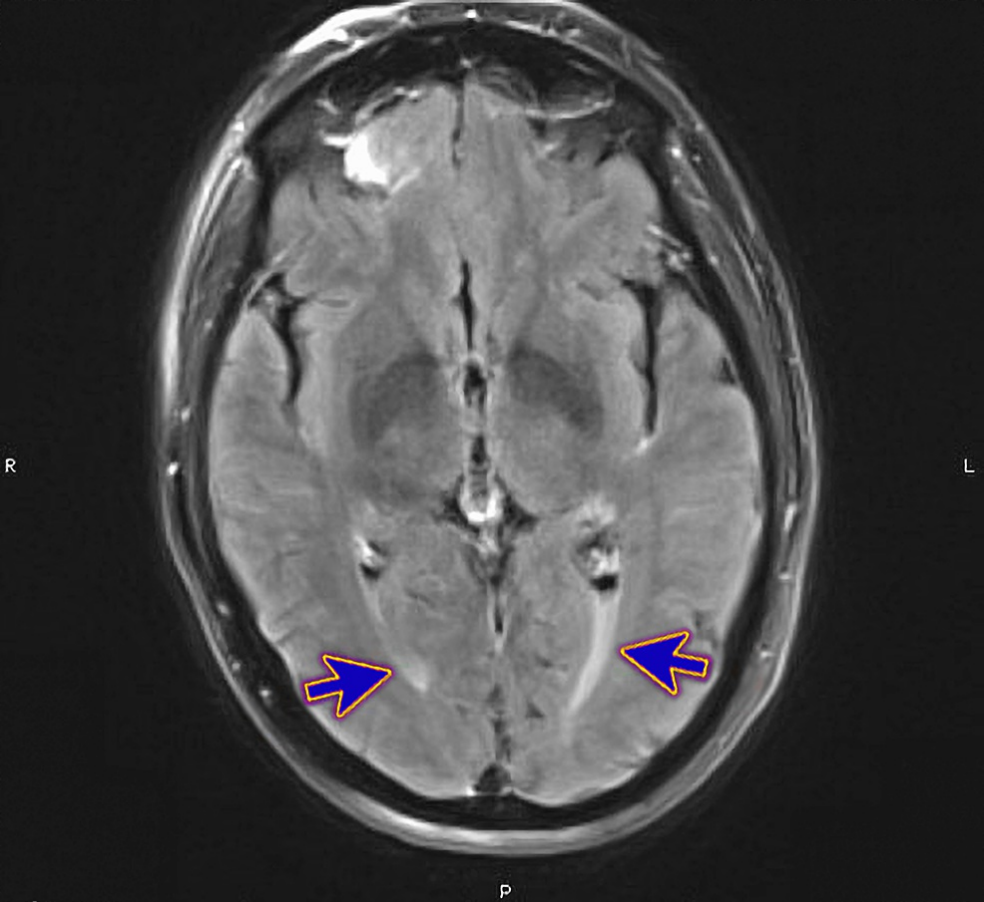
Magnetic Resonance Imaging (MRI) of the brain showing interval resolution of the sulcal and ventricular enhancement with resolved pus/ventriculitis (blue arrows)
